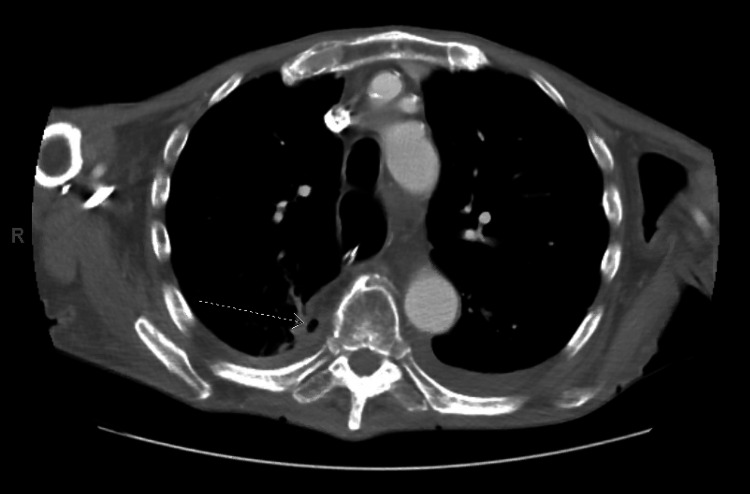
Axial CT of the chest with contrast showing a GBF (dotted arrow) GBF, gastrobronchial fistula
